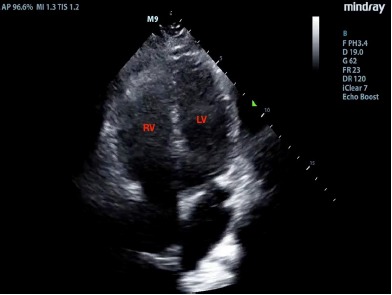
Apical four-chamber view of the heart, demonstrating an enlarged right ventricle (RV), greater in size than the left (LV).
